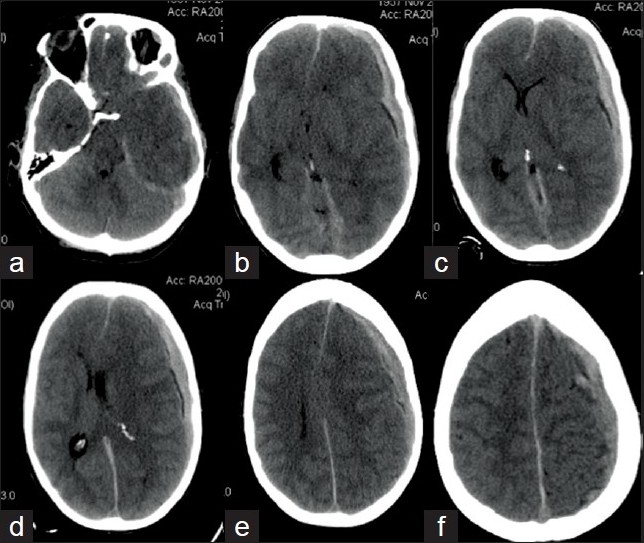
(a–f) Head CT showing left acute fronto-temporo-parietal subdural hematoma with mass effect, midline shift and transtentorial herniation
